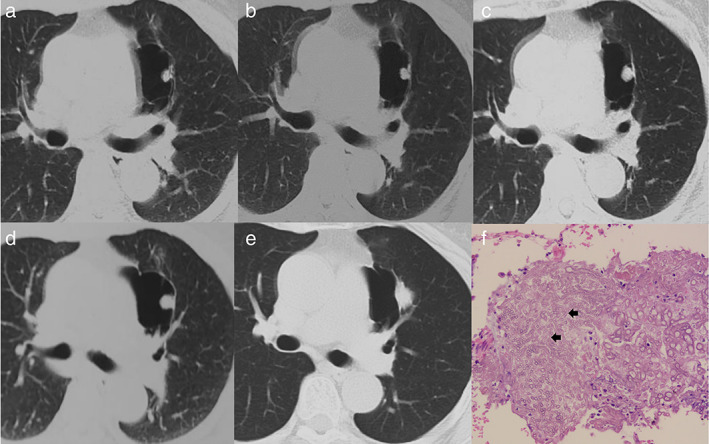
(a)–(d) Chest CT images of the patient in 2016, 2017, 2018, and 2019. (e) The lesion was significantly larger compared with its size in 2016. (f) Postoperative pathology indicated focal alveolar epithelial hyperplasia complicated by high levels of acute and chronic inflammatory cell infiltration, fibrosis, and hyphae (black arrow) in the lesion
